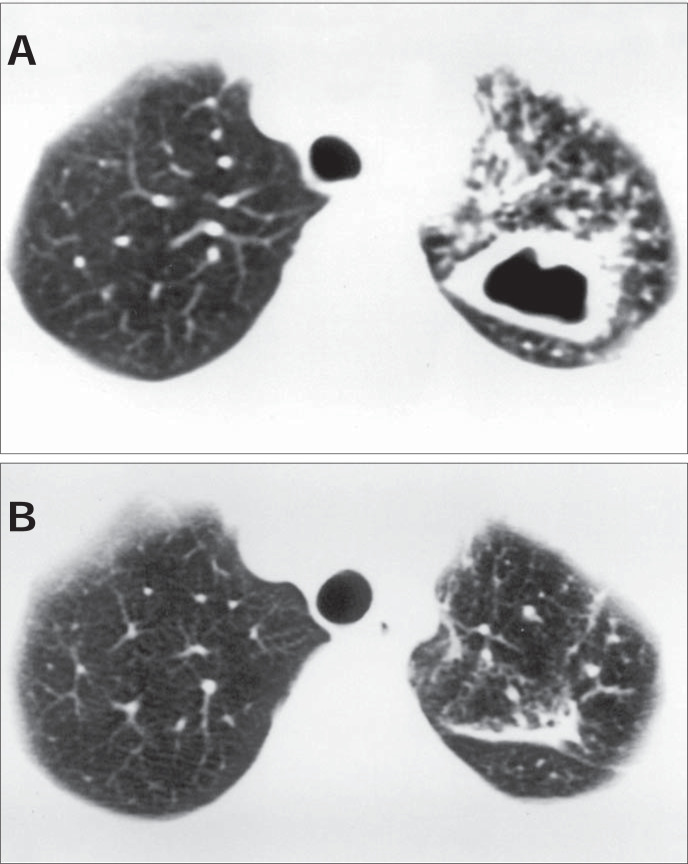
(A) Computed tomography at the start of treatment: thick-walled cavity in the left lung. (B) Computed tomography post-treatment, for the same patient: there is a band in the place where a cavity had been observed at the start of treatment.
